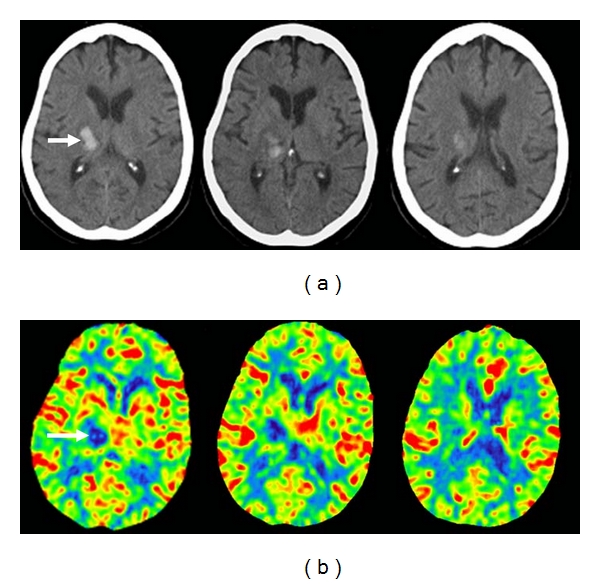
75-year-old woman presented with left hemiparesis and headache. (a) Axial unenhanced CT images show a deep right thalamic hemorrhage (arrow) sparing the caudate nucleus. (b) Cerebral blood flow images show an area of decreased flow matching the area of the hematoma. There are no underlying features to suggest that this is a hemorrhagic infarct.
